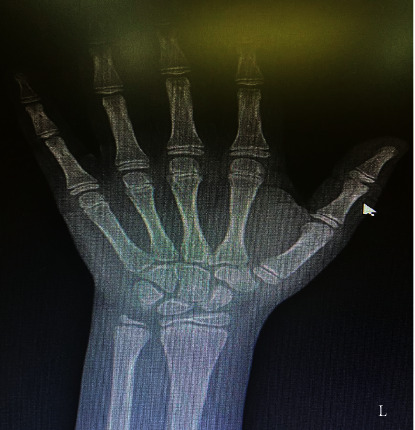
Left wrist X-ray showing a bone age of 10 years in contrast to chronological age of 13.6 years. Bone age was determined using Greulich and Pyle's radiographic atlas of skeletal development of the hand and wrist [6].
